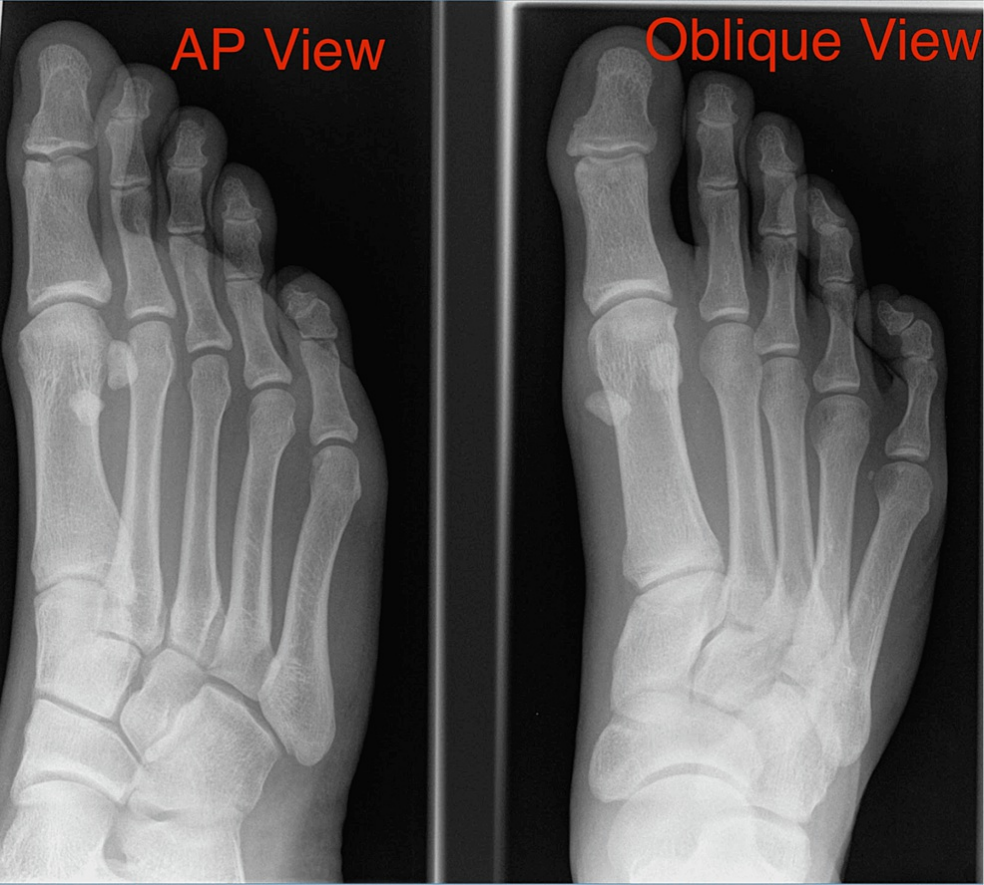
X-ray of the right foot showing subluxation of the first metatarsophalangeal joint in oblique view X-rays of a 32-year-old male patient with hyperextension injury of the great toe. Oblique X-rays showing subluxation of the first metatarsophalangeal joint suggestive of a turf toe injury.
